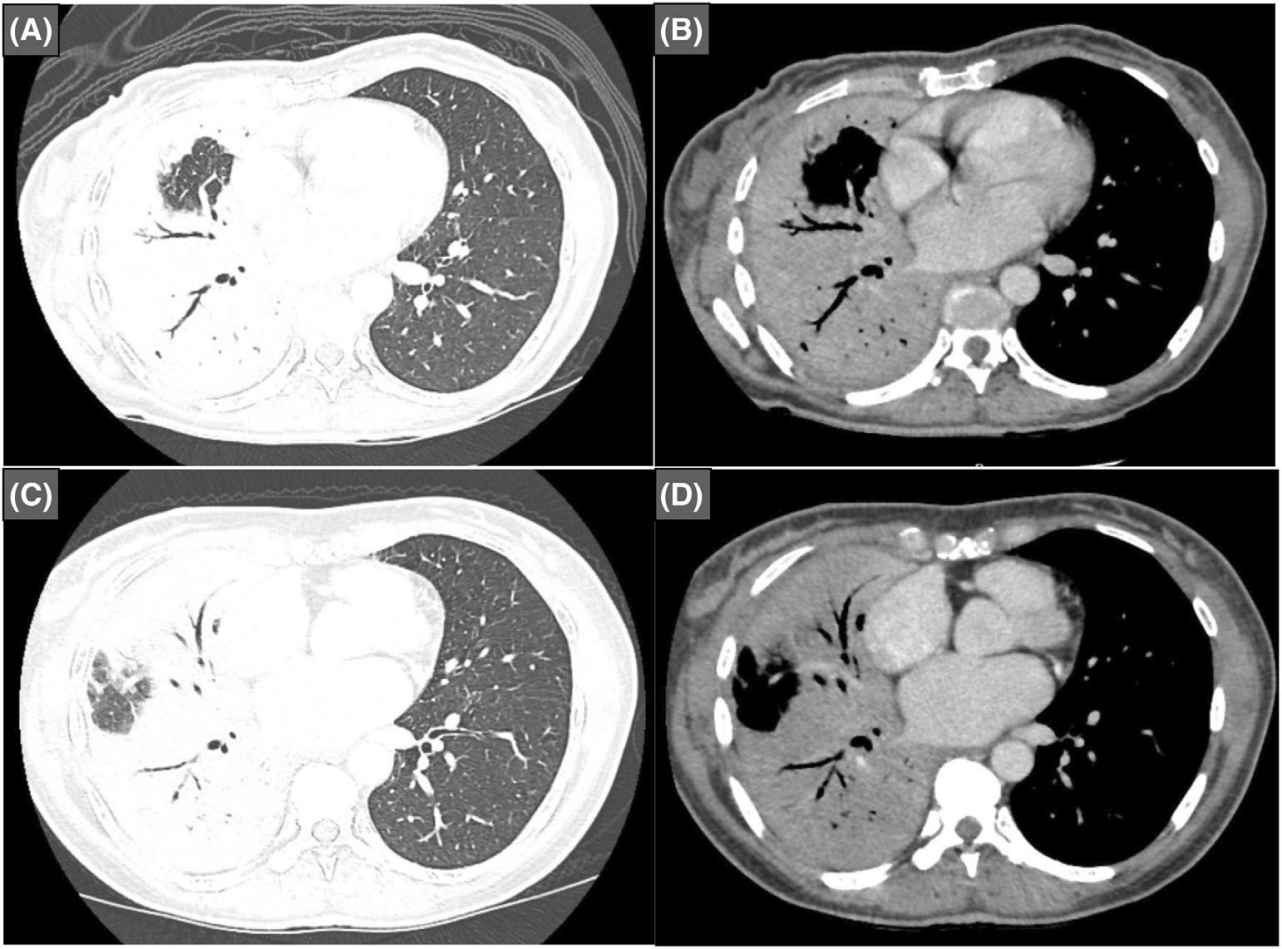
(A, B) Computed tomography (CT) of the thorax done approximately 2 months after initial presentation demonstrating persistent right lung consolidation. (C, D) Repeat CT thorax 6 months after the initial CT again revealing a persistent right lung consolidation, showing no response towards anti‐tuberculous medications.
